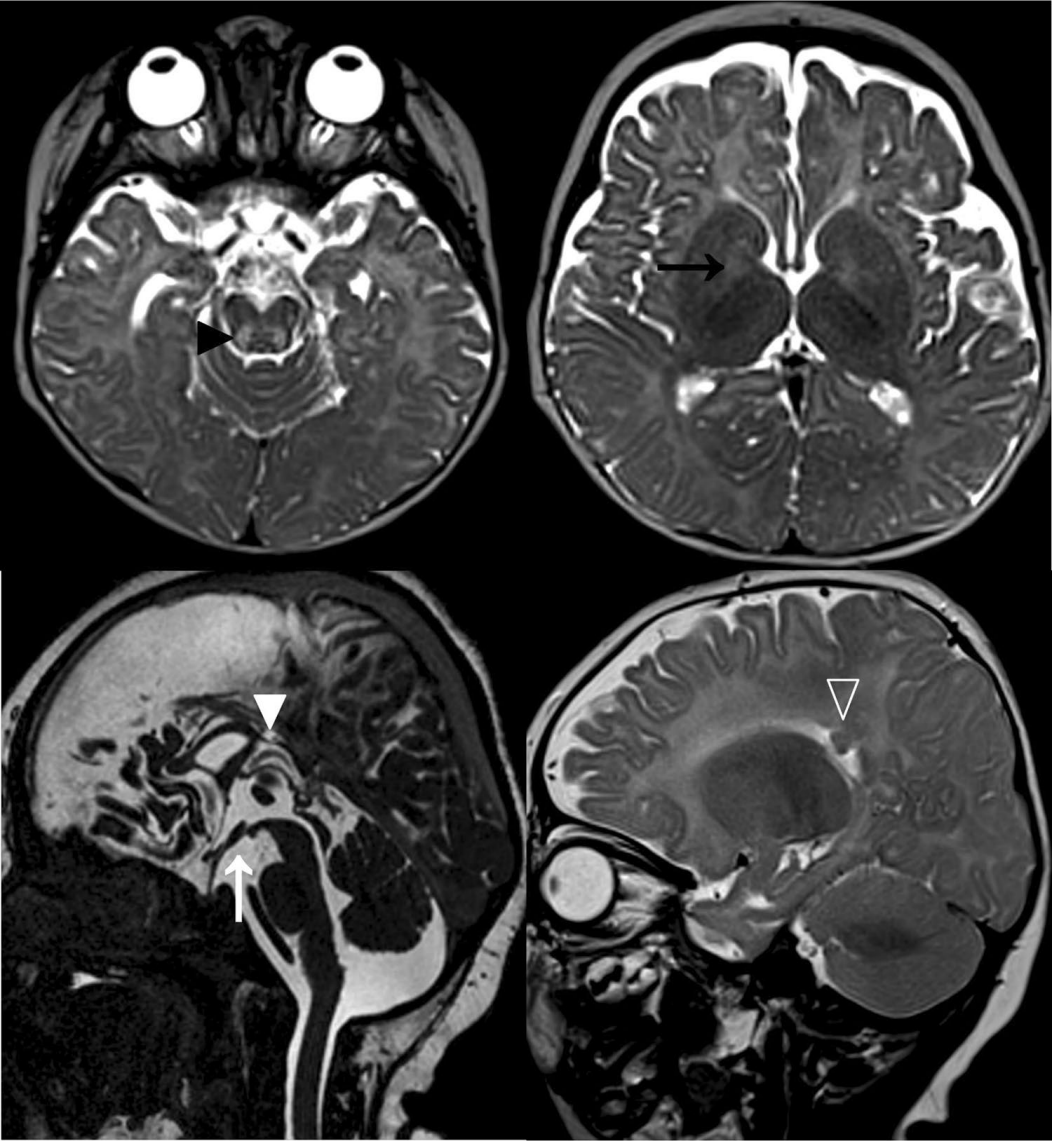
MRI findings in individual 2 at 8 months of age. Myelination appears slightly delayed for age with arborisation of the frontal white matter not yet present. Increased T2 signal intensity of the decussation of superior cerebellar peduncle (black arrow head) and medial pallidi (black arrow) is noted. At the age of 5 months, these lesions had shown restricted water diffusion, suggestive of cytotoxic edema. The body of corpus callosum is hypoplastic (white arrow head) and bilateral subependymal heterotopias are noted at this level (open arrow head). In addition, hypothalamic adhesions are present (white arrow)
